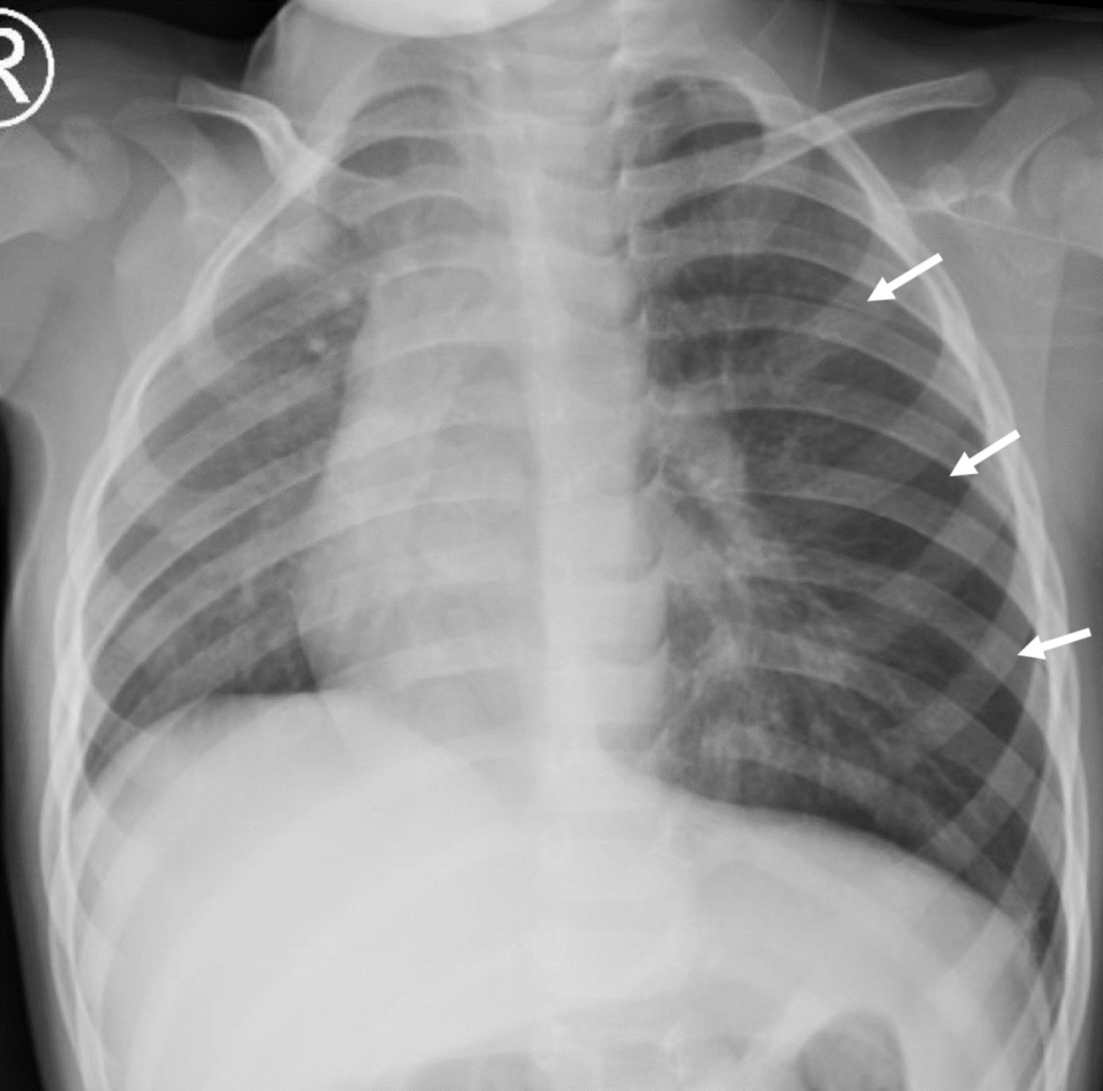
Chest X-ray on day six demonstrated stable left-sided pneumothorax; white arrows outline the lateral aspect of the lung
